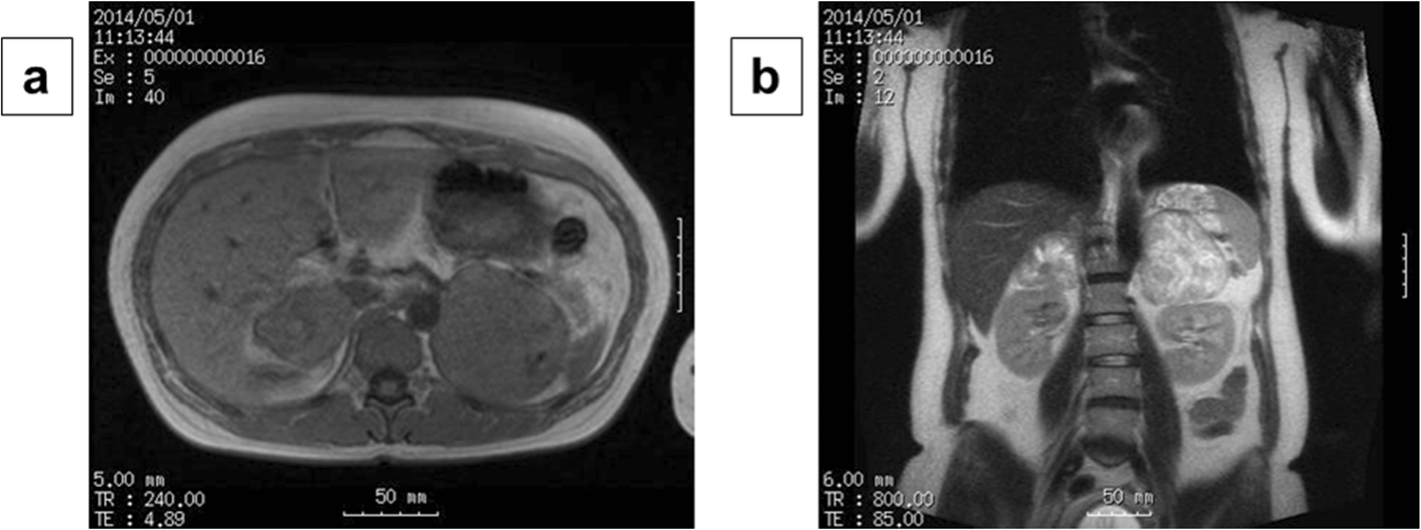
MRI findings: MRI shows bilateral adrenal masses with central necrosis. The right mass was 4.9 × 4.4 × 4.2 cm and the left mass was 7.3 × 6.1 × 7.5 cm, which were compatible with bilateral pheochromocytoma. A tumor exhibiting relative non-uniform low signals on T1 MRI was observed in both adrenal glands. Transverse plane (a). Coronal plane (b).
